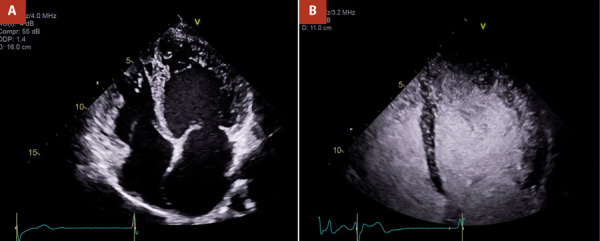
Echocardiogram in apical four-chamber view without (A) and with contrast (B) showing left ventricular hypertrabeculation, dilatation, and mildly impaired left ventricular systolic function.
